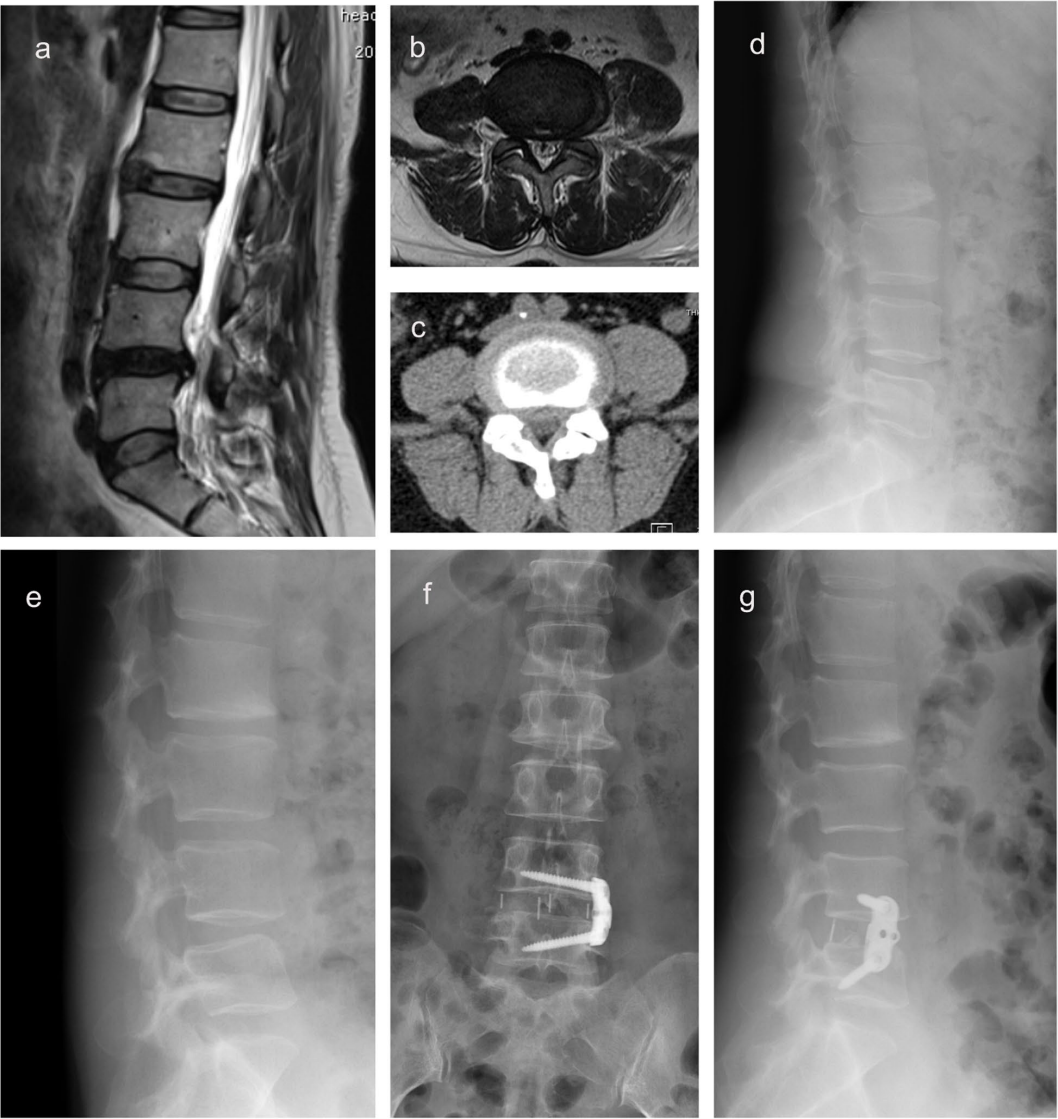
Preoperative and postoperative radiographs of a case with lumbar spinal stenosis. a Preoperative sagittal T2-weighted MRI image. b Preoperative axial T2-weighted MRI image indicated lumbar stenosis at L4-5. c Preoperative axial CT showed that lumbar stenosis at L4-5. d, e Preoperative flexion-extension stress lateral radiographs. f, g Anteroposterior X-ray of lumbar spine after XLIF
